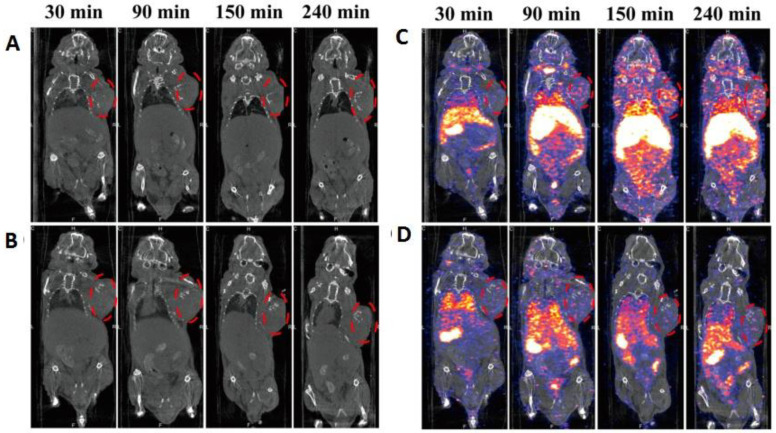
(A,B) *In vivo* CT and (C,D) SPECT/CT images of a xenografted tumor model after intravenous injection of {(Au^0^)_6_-G2-DTPA (^99m^Tc)-PEG-FA} DENPs (A,C, respectively) and ({(Au^0^)_6_-G2-DTPA (^99m^Tc)-mPEG} DENPs) (B,D, respectively). High uptake in the liver, spleen, and tumor is visible. Reprinted with permission from [40], with permission from American Chemical Society, 2016.
